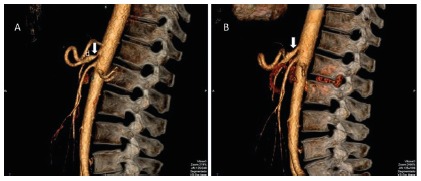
A) Computed tomographic angiography of the abdomen demonstrating
